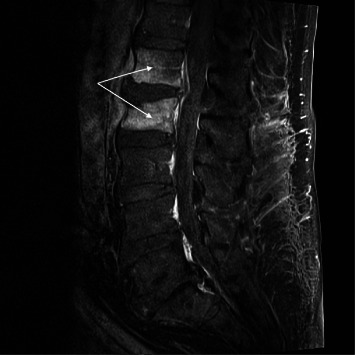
Second MRI sagittal (30.12) T1 fat sat injected. White arrow showing contrast-enhancing inflammatory changes in L1 and L2 vertebrae with preserved intervertebral disc.
